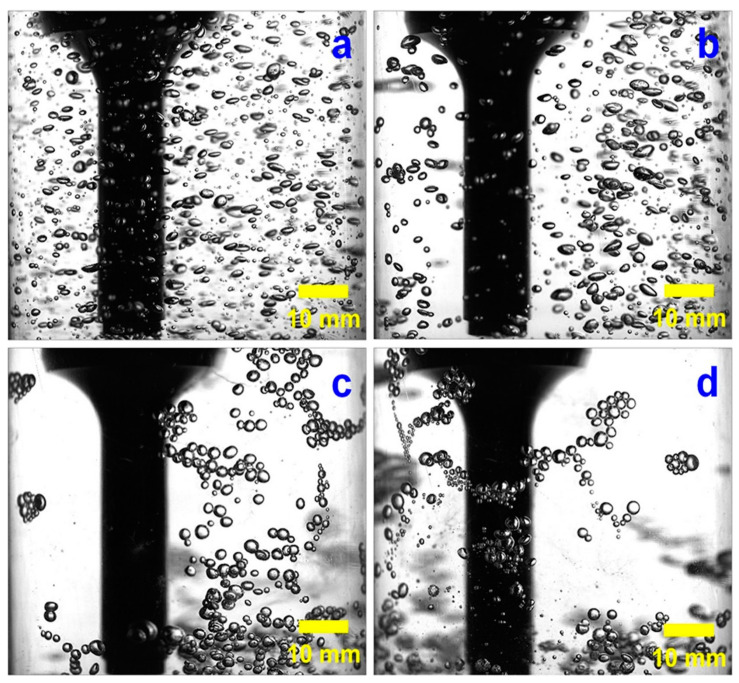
Images of the effects of ultrasonic power on bubble aggregates at 3 s of ultrasonic treatment ((a) 0 W; (b) 20 W; (c) 110 W; (d) 200 W) [94].
